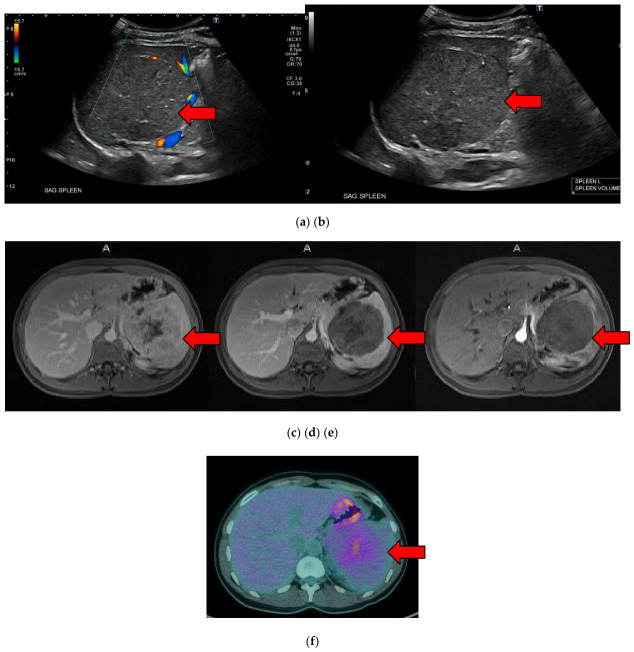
Post-traumatic pseudotumor. (a,b) Ultrasound demonstrates a well-circumscribed splenic mass with some peripheral vascularity upon Doppler interrogation. (c–e) MRI demonstrates gradual enhancement of the central fibrous components on post-gadolinium T1 fat-suppressed MRI. (f) PET/CT demonstrates mild FDG avidity, greater than the background liver.
